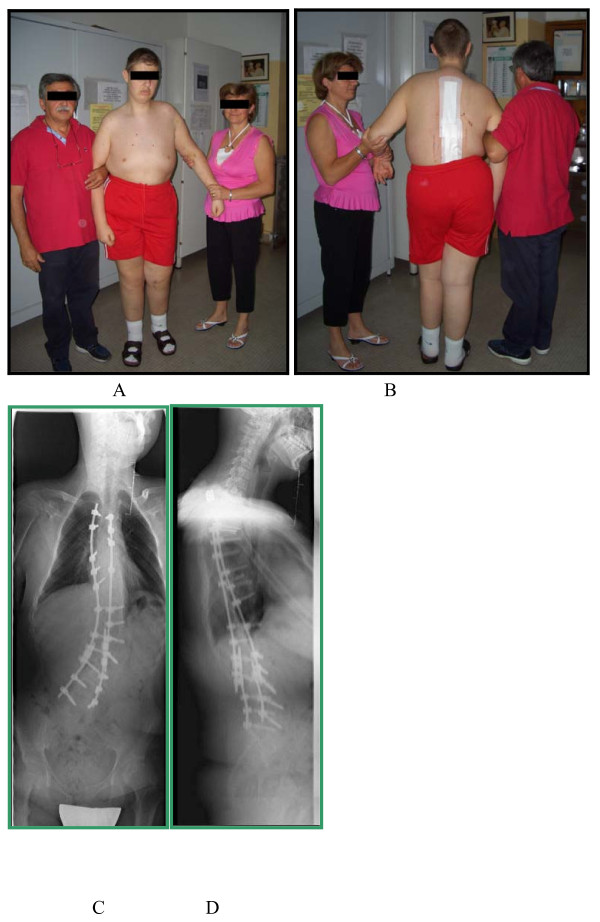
Clinical and radiographic picture at a 6-month follow-up. After performing revision surgery and extending arthrodesis to L5, correction was stable.
